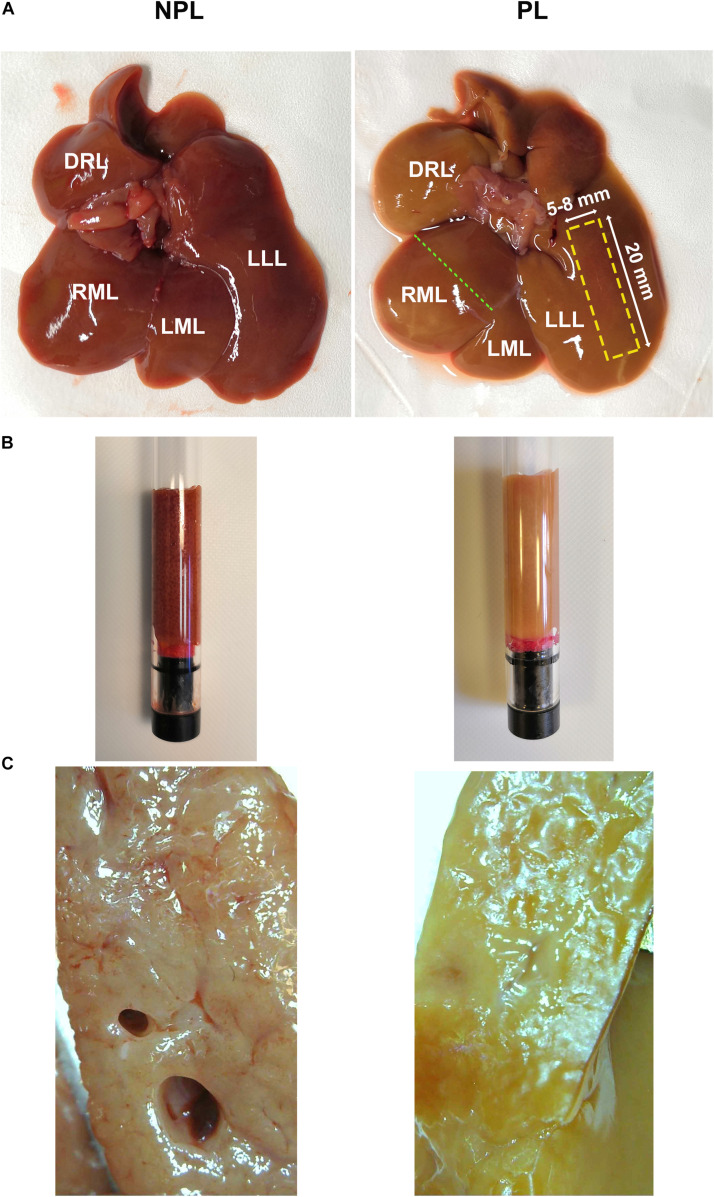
(A) Photograph of fresh non-perfused livers (NPLs, left) and perfused livers (PLs, right); a rectangular area marked by a dashed yellow line indicates the site from which the sample for MRI was taken. The green dashed line indicates the position of the cross-sectional area shown in (C). (B) Sample tubes containing liver tissue obtained from NPL and PL for DWI and MRE. (C) cross-sectional area of the liver showing more blood in the NPL sample (left) than in the PL sample (right). NPL, non-perfused liver; PL, perfused liver; LLL, left lateral lobe; LML, left middle lobe; RML, right middle lobe; DRL, dorsal right lobe.
